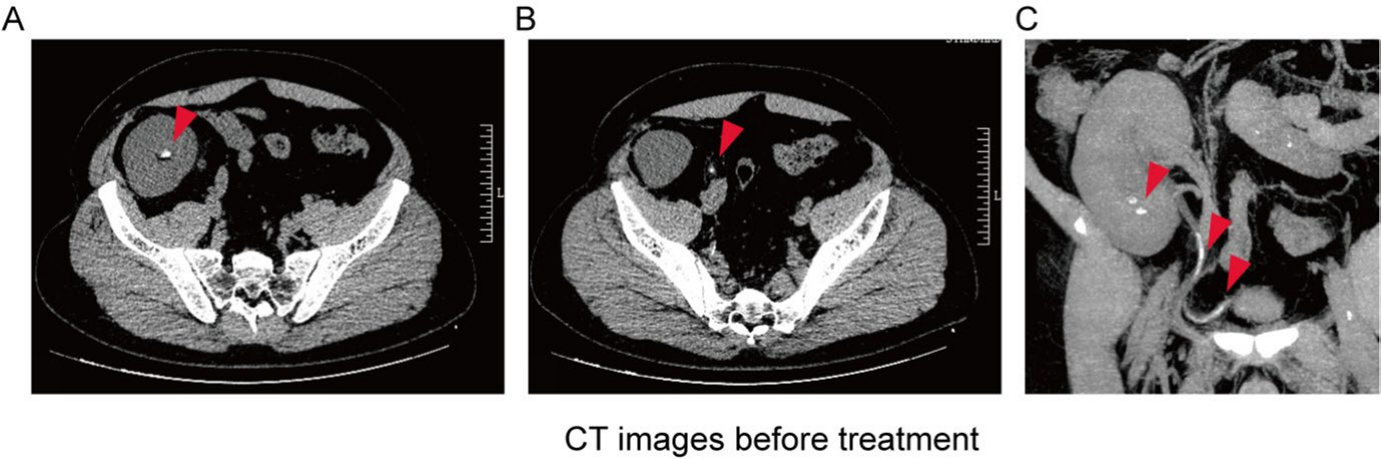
A CT image showed that the patients had a transplanted kidney stone before treatment. B and C. CT image showed that the patients had ureteral calculus before treatment
